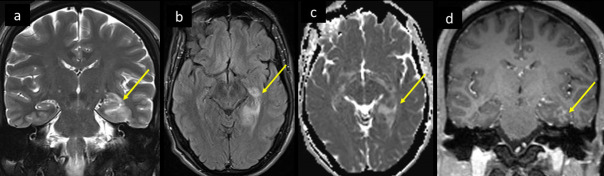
34-year-old male, presented with to the ED with 4 days of mild fever and flu like symptoms, with increasingly severe headache, photophobia, and dizziness for 2 days. He tested positive for COVID, and all his CSF work-up was negative. MRI brain coronal *T*_2_ (a) shows mildly swollen bright hippocampus and medial temporal lobe, axial FLAIR-*T*_2_ (b), ADC (c) and cororonal *T*_1_ post-i.v. contrast, shows corresponding bright signal, facilitated diffusion, and patchy post-contrast enhancememnt respectively (arrow in a–d). ADC, apparent diffusion coefficient; CSF, cerebrospinal fluid; FLAIR, fluid attenuated inversionrecovery
